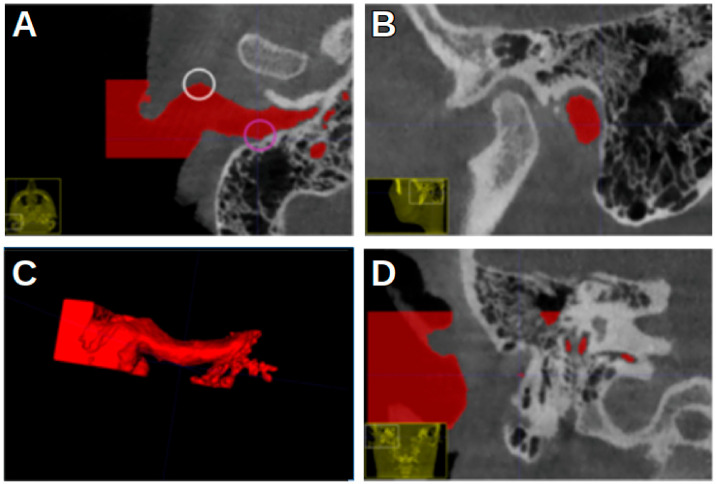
An ITK-SNAP window with a CBCT scan of a left acoustic meatus with red segmentation. (A) Axial view. (B) Sagittal view. (C) Three-dimensional segmentation. (D) Coronal view. White circle: the most anterior point of the segmentation. Pink circle: the most posterior point.
